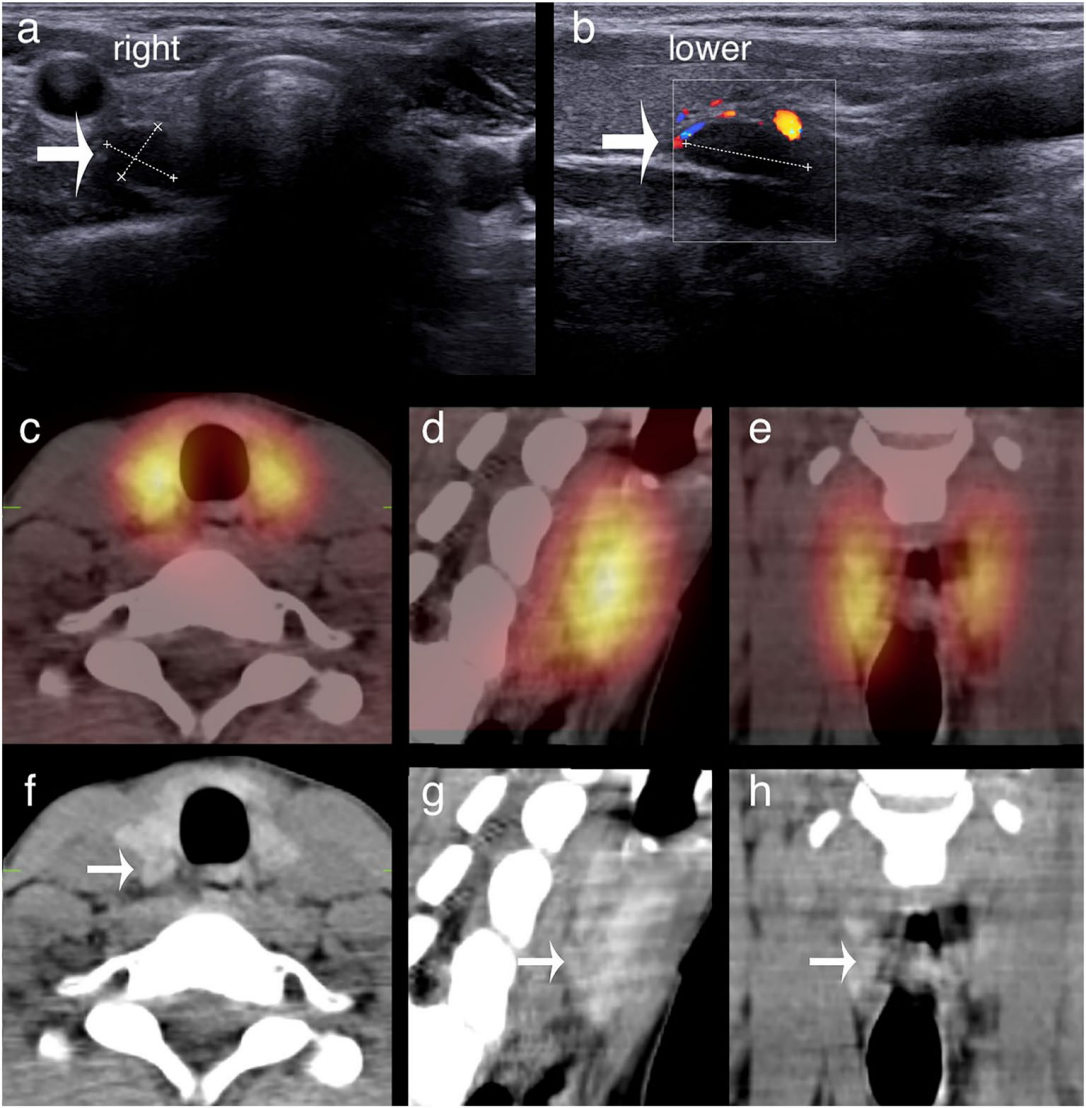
Upper parathyroid adenoma localised inferiorly by ultrasound and [^99m^Tc]pertechnetate/MIBI SPECT/CT. (a,b) Transverse and longitudinal ultrasound sections. In (b), only the lower part of the thyroid is seen. (c–e) Fused [^99m^Tc]pertechnetate/MIBI SPECT/CT axial, sagittal and coronal slices. (f–h) Corresponding CT slices. The adenoma (arrows) is seen adjacent to the inferior third of the right thyroid lobe but was surgically revealed to be an upper adenoma.
